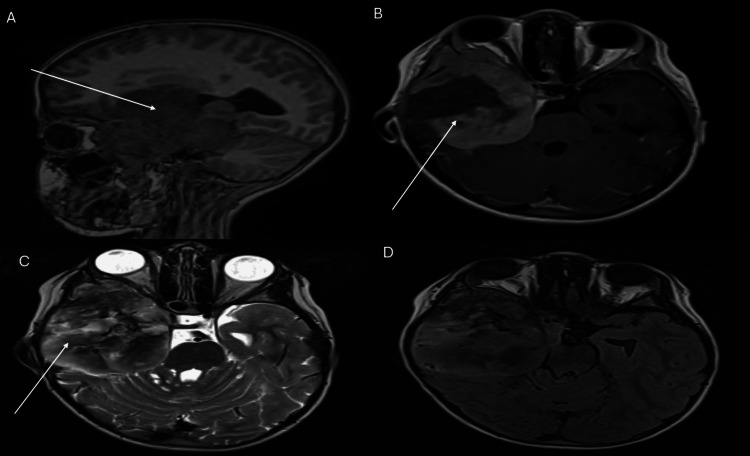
MRI brain imaging. A voluminous right temporal lesion with an extra-axial appearance is observed, showing low signal on T1 (A), high signal on T2 (C), and heterogeneous enhancement following contrast injection (B). Isointense on FLAIR sequence (D). The mass measures 60 mm in transverse diameter and 78 mm in anteroposterior diameter. It occupies the entire right temporal fossa, extending into the cavernous sinus medially, with a mass effect on the midbrain posteriorly and the third ventricle superiorly. Anteriorly, the mass invades the posterior part of the ocular globe, causing exophthalmos MRI: magnetic resonance imaging; FLAIR: fluid-attenuated inversion recovery
